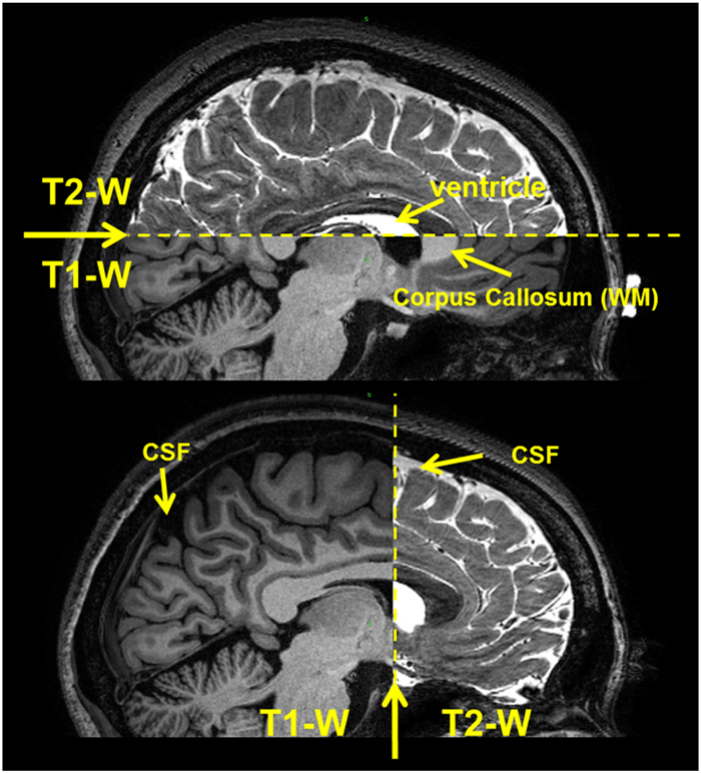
T1- and T2-weighted MRI registration. Sagittal view of the registered and integrated T1- and T2-weighted MRIs. The contrast between WM, e.g., corpus callosum, and GM signals is higher in T1, while the CSF, e.g., ventricle, is enhanced in T2. The tissue segmentation was performed by reaping the benefits of both MRI datasets.
